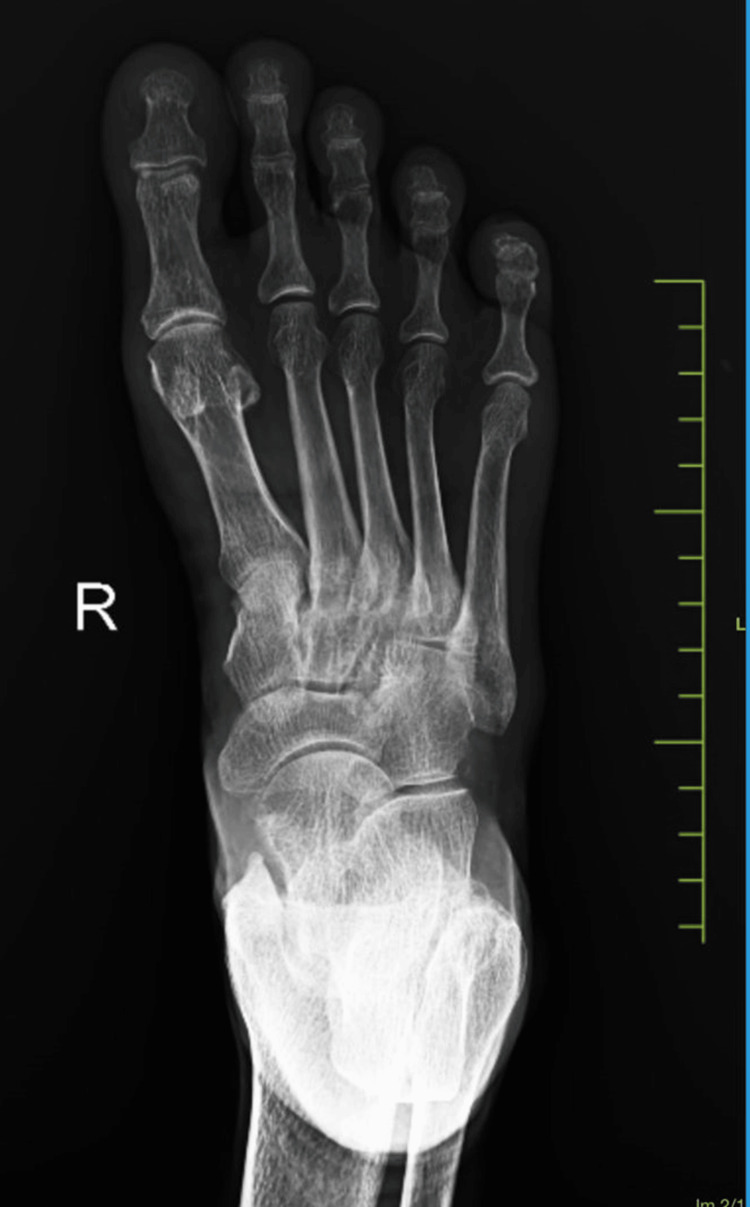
X-ray image of the foot (anteroposterior view)
